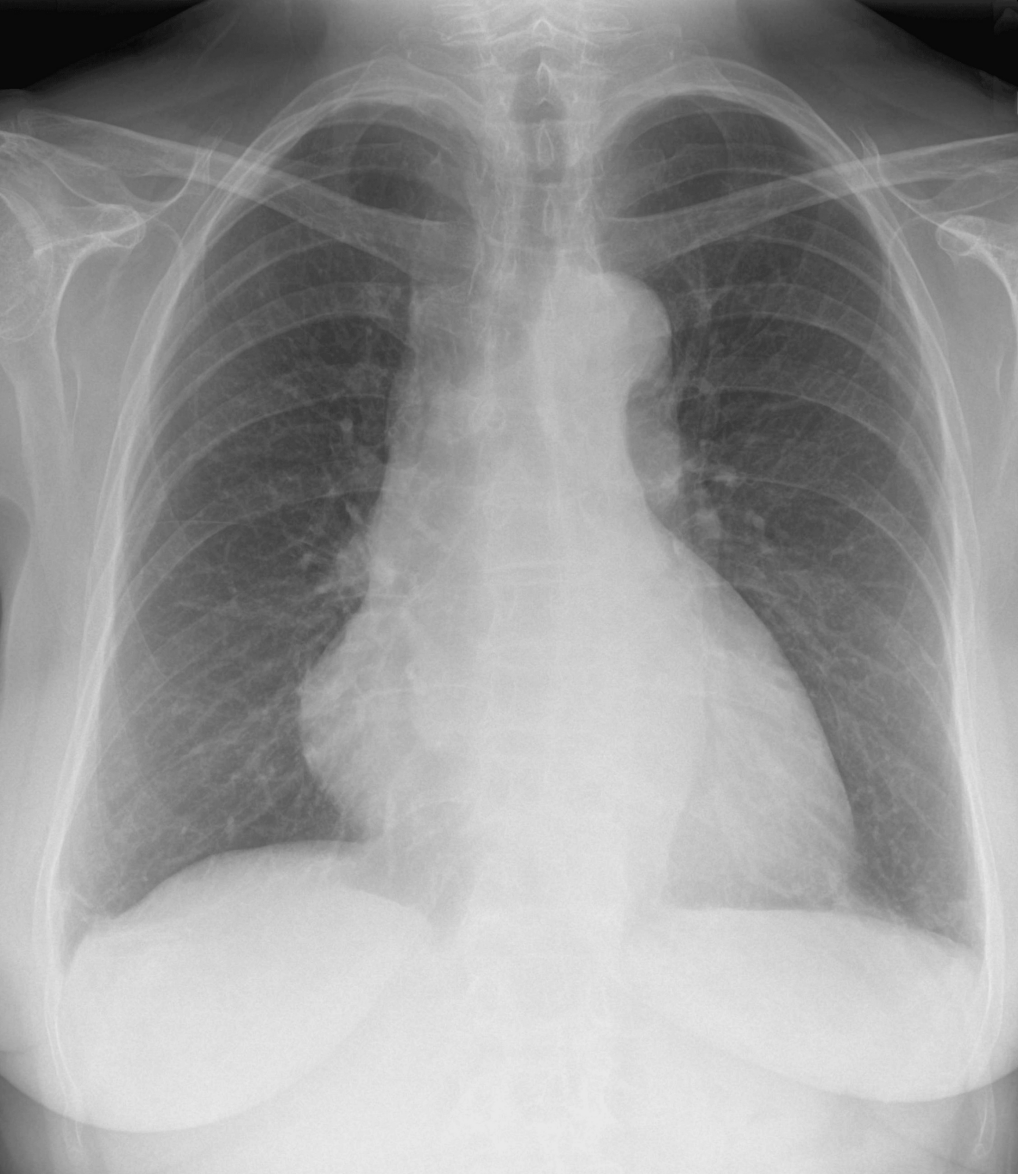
Chest X-ray after 2 weeks showing resolution of pleural effusion
